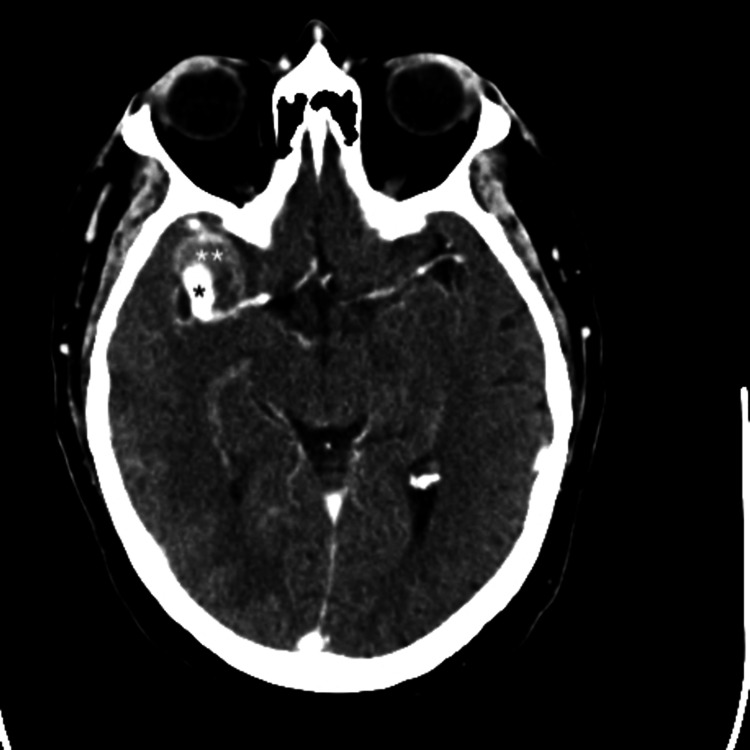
Axial CT scan with contrast shows a subarachnoid hemorrhage secondary to the rupture of a right partially thrombosed MCA bifurcation aneurysm. The ischemic stroke is not visible on this scan. *Circulating part of the MCA aneurysm. Thrombosed part of the MCA aneurysm. MCA, middle cerebral artery
